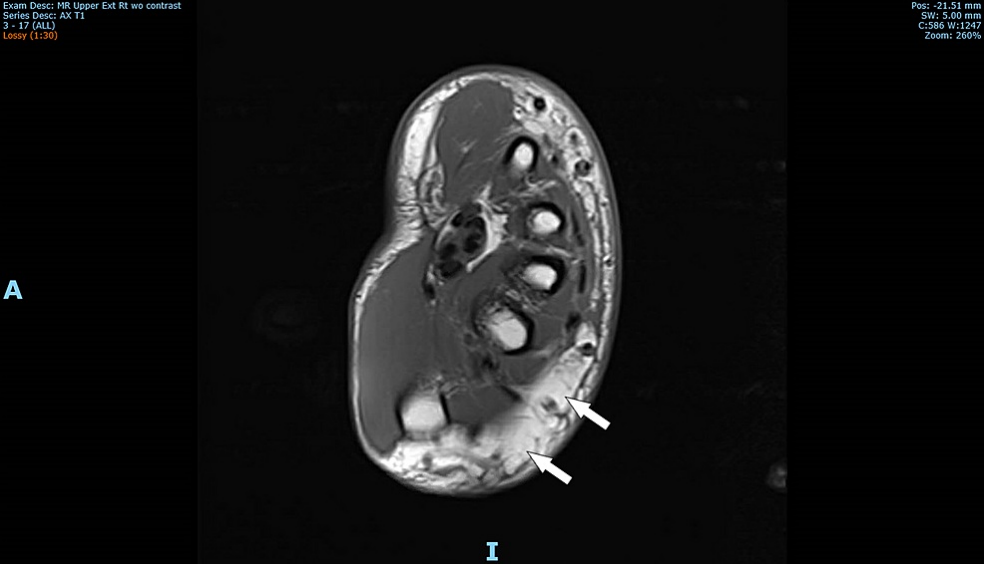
Axial MRI of right upper extremity revealing marked subcutaneous edema
